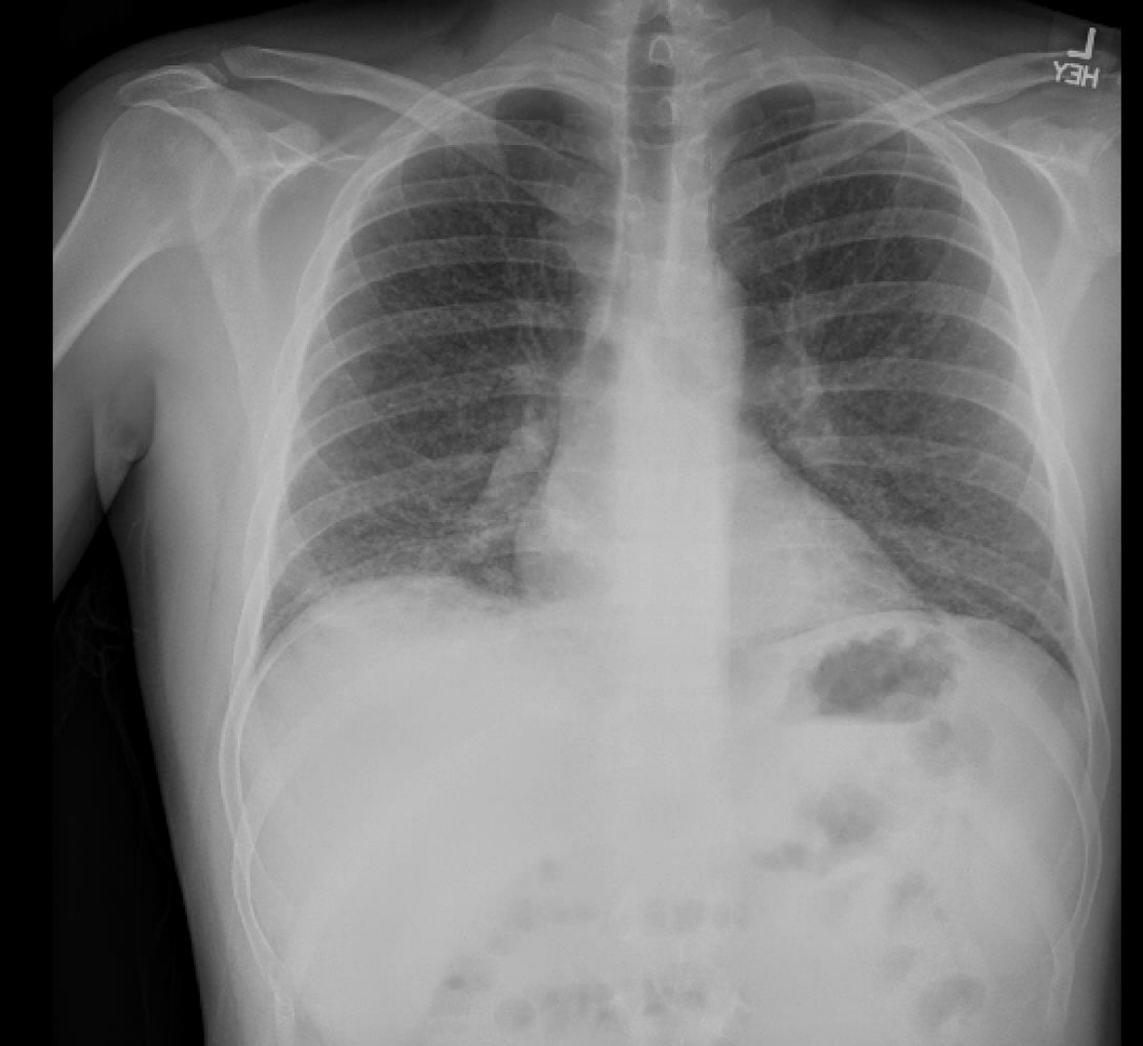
Chest X-ray in the emergency department. Image shows increased interstitial markings with suggestion of a right lower lobe more focal consolidation.
